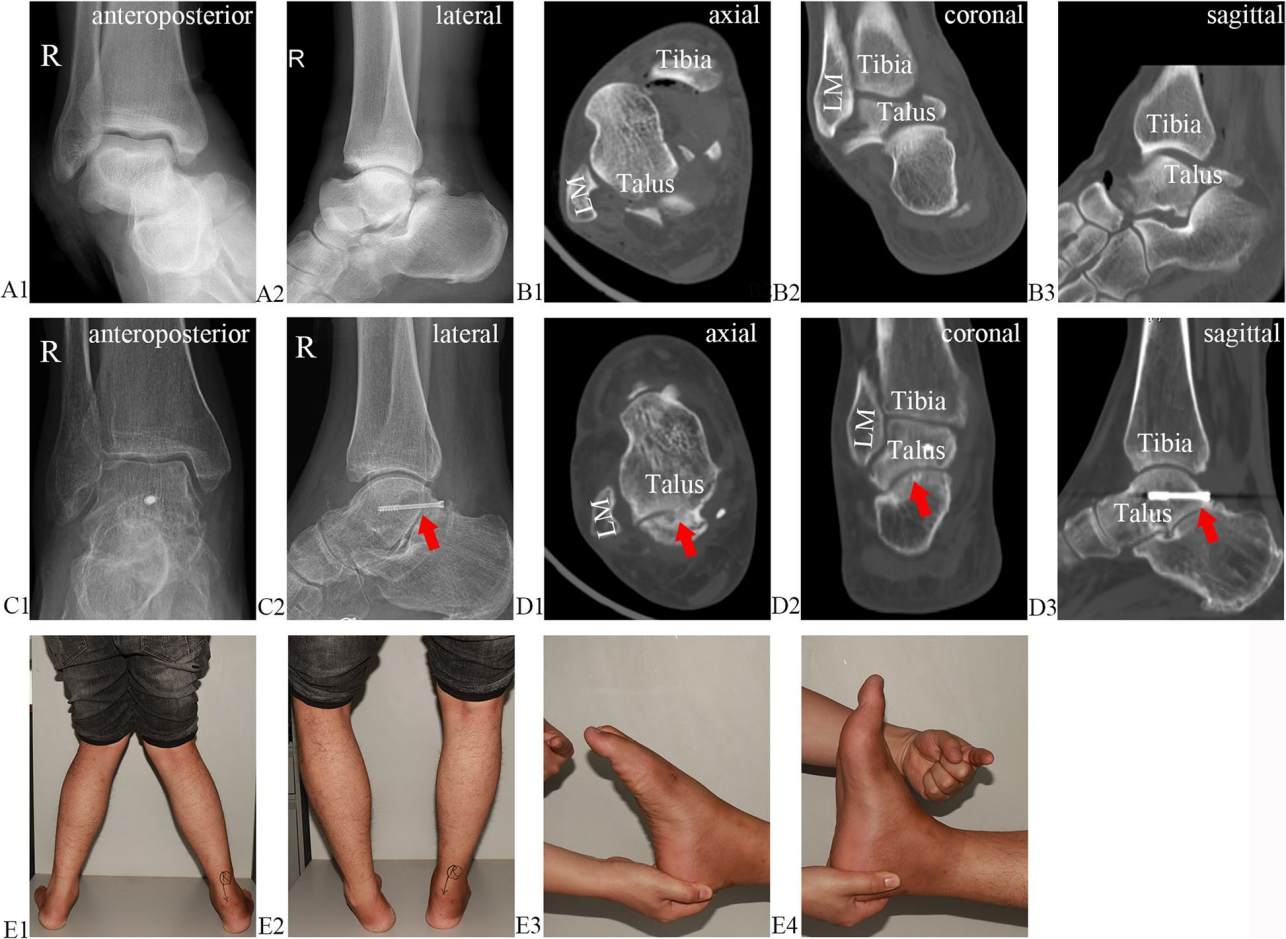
X-ray radiography and CT results before (A1–2 and B1–B3, respectively) and 18 months after (C1–2 and D1–D3, respectively) the operation. LM: lateral malleolus. Red arrow: sub-talar osteoarthritis. Functional results 18 months after the operation (E1–E4)
